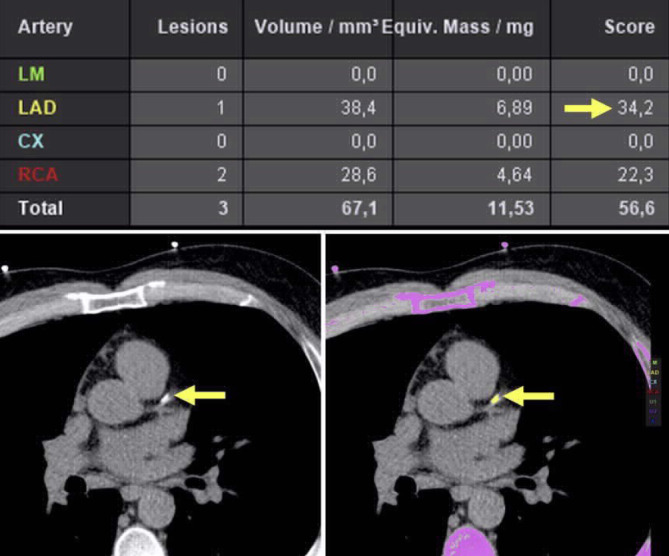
## Hochrisikoplaques und CT-Koronarangiographie als prognostische Langzeitparameter
